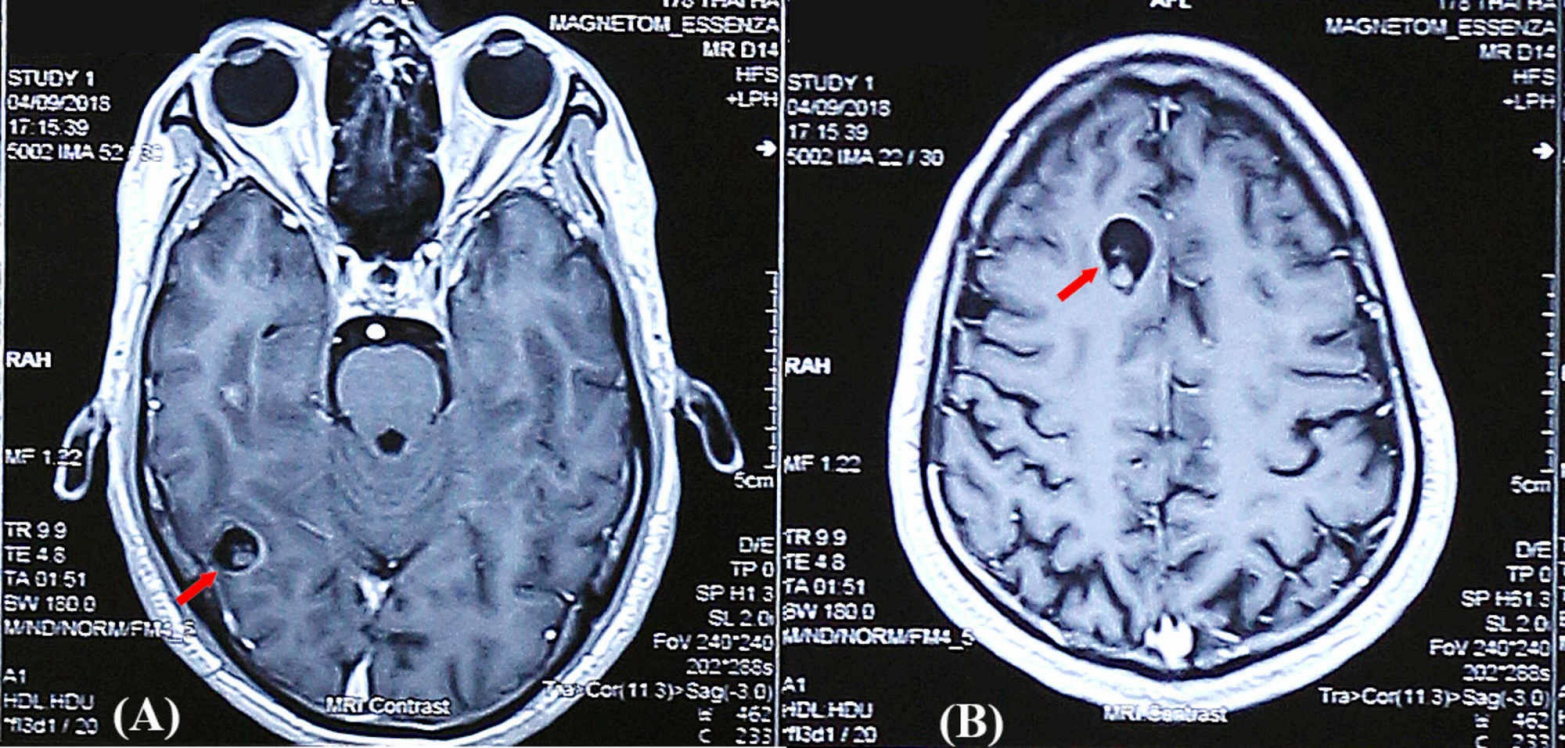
MRI findings of case 1 (axial T1 weighted images) The axial T1 weighted image after contrast showed a ring-enhancing cystic lesion with a well-defined border in the right occipital lobe. The diameter of this lesion was 11 mm. A scolex was demonstrated as a small image associated with a hyperintense cyst wall (A). A similar lesion was found in the right frontal lobe with 10 mm in diameter (B) MRI: magnetic resonance imaging
